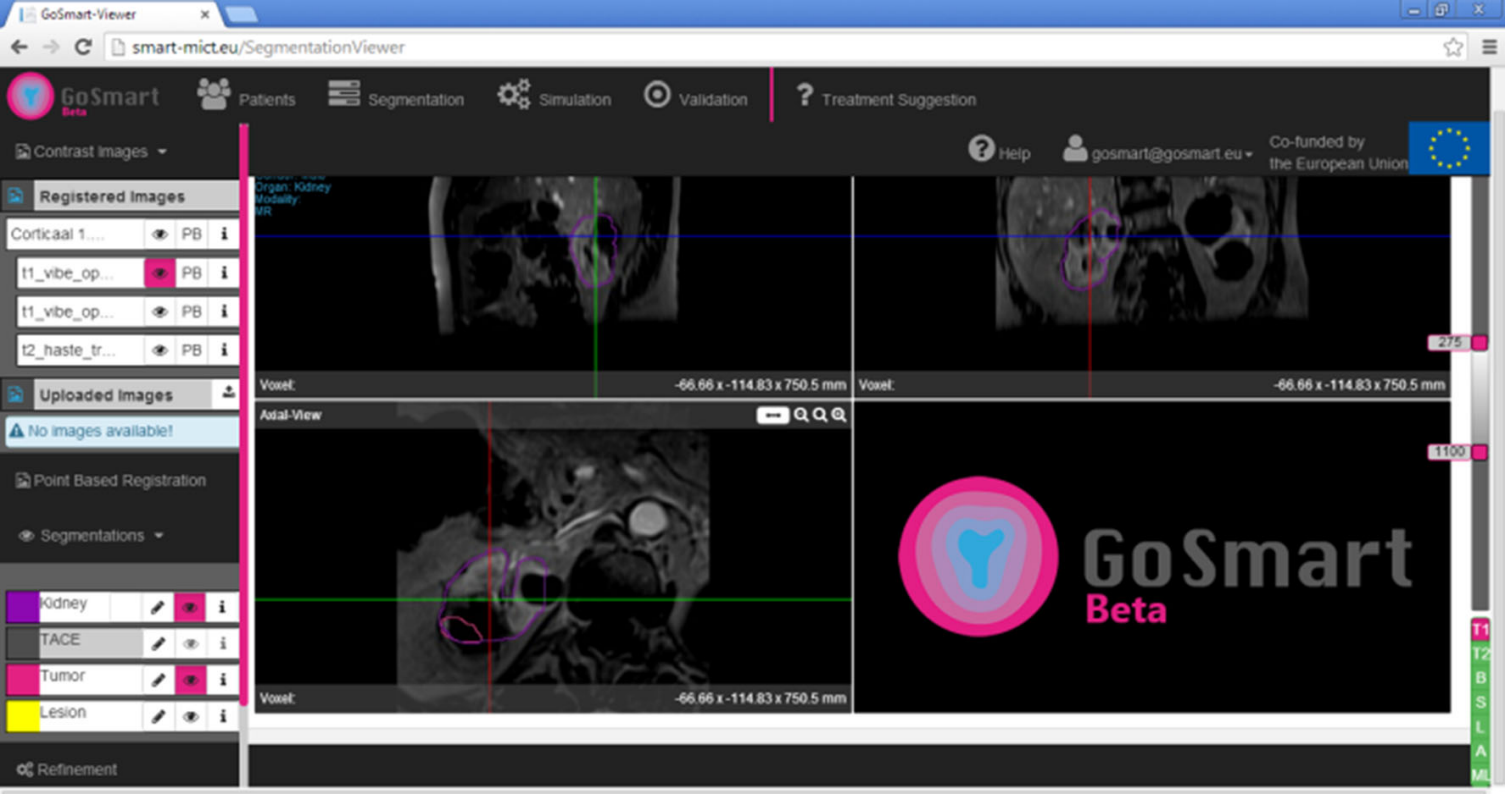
Web environment viewer showing MRI in three orthogonal planes. Available image series and tools are shown in bars on the left
